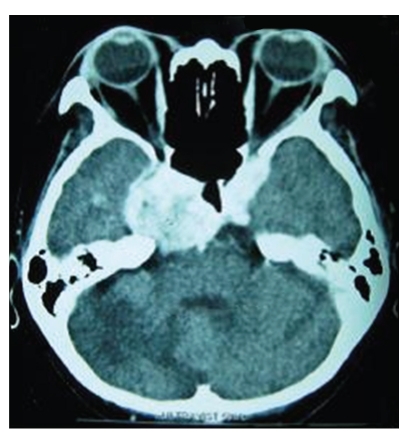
Cranial computed tomography (CT) scan showing a 4 cm enhancing extradural EBV-SMT at the medial aspect of the right tentorium cerebelli, with erosion of the petrous apex and extending into the right optic canal, prepontine cisterns and encasing right carotid (cavernous portion) artery (image reproduced with permission from [13]. The AIDS Reader, UBM Medica).
